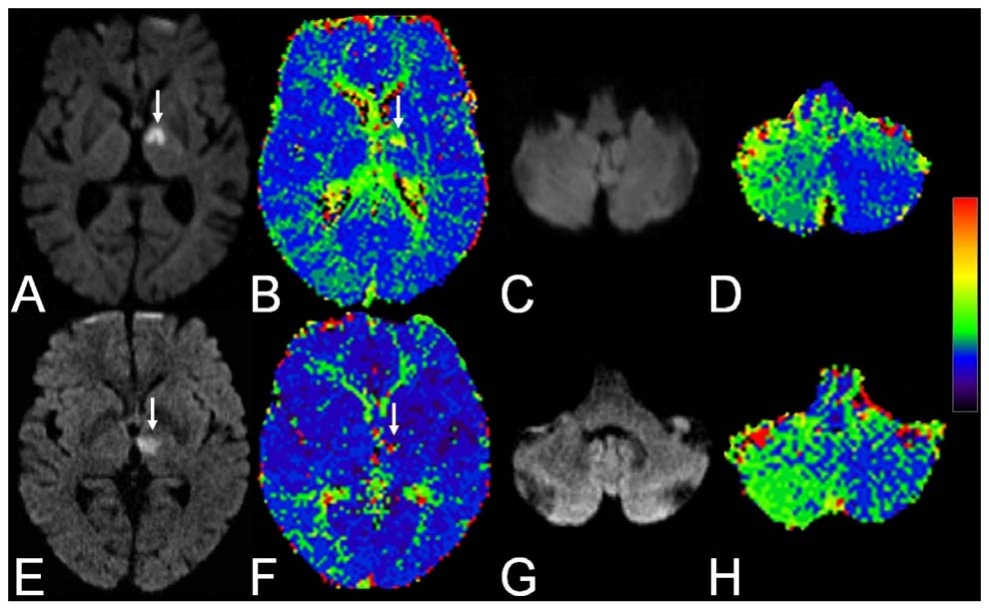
Two examples of crossed cerebellar diaschisis. 1. A 75-year-old female patient with reduced vigilance, dysarthria, and right-sided hemihypaesthesia: *A* Trace diffusion-weighted image shows an acute ischemic lesion (arrow) in the territory of the left tuberothalamic artery with *B* matching hypoperfusion (arrow) on the time to peak (TTP) map. *C* In the cerebellum trace DWI is unremarkable while *D* TTP demonstrates hypoperfusion in the contralteral cerebellar hemisphere. 2. A 65-year-old female patient with right-sided hemiparesis: *E* Trace DWI shows an acute ischemic lesion (arrow) in the territory of the left paramedian artery with *F* minor hypoperfusion (arrow) on TTP. *G* In the cerebellum trace DWI is unremarkable while *H* TTP demonstrates hypoperfusion in the contralateral cerebellar hemisphere.
